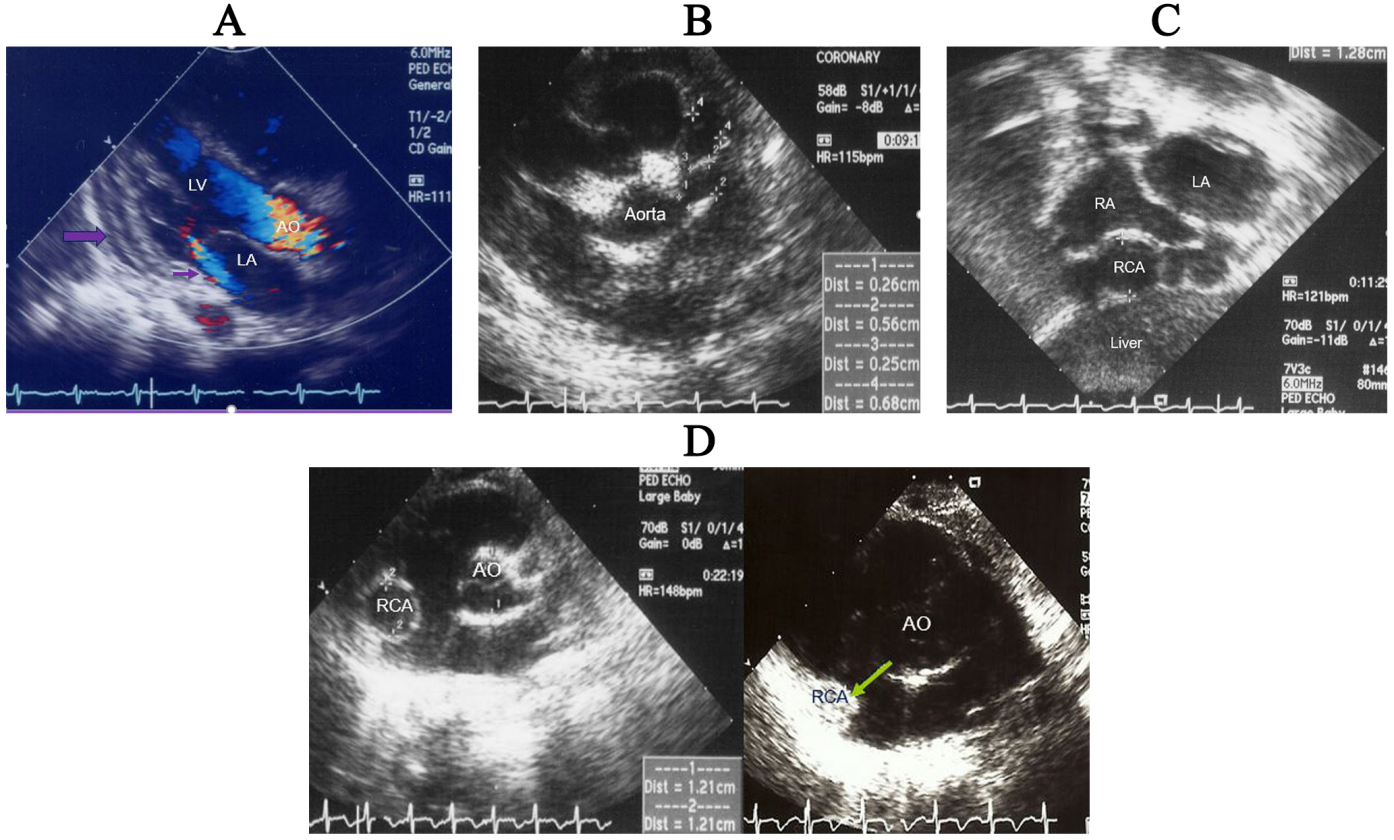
Echocardiographic images of a 3-month-old infant with KD.
